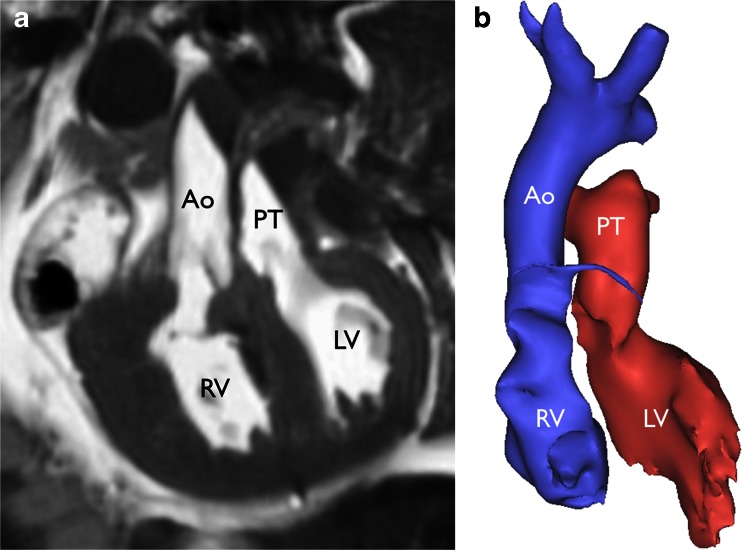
Neonatal cardiac postmortem MRI in a 1-day-old neonate with transposition of the great arteries. Coronal T2-weighted image (a) and 3-D volume-rendered reconstruction (b) show the aorta (*Ao*) arising from the right ventricle (*RV*) and the pulmonary trunk (*PT*) arising from the left ventricle (*LV*). Reproduced with permission [14]
